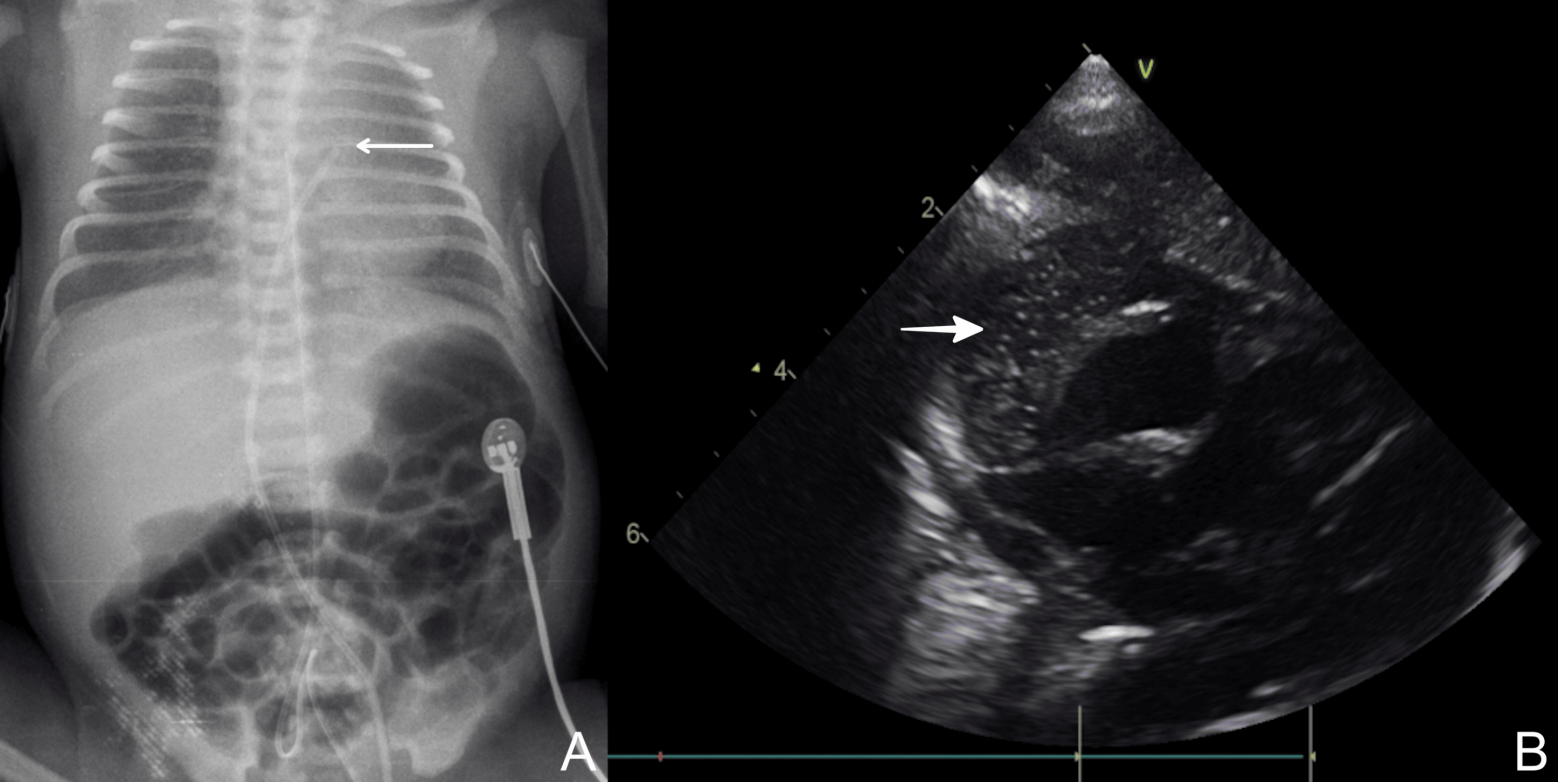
Case 1: (A) X-ray showing a high position of UVC (arrow); (B) Echocardiography showing the pericardial effusion (bold arrow). UVC: umbilical venous catheter
